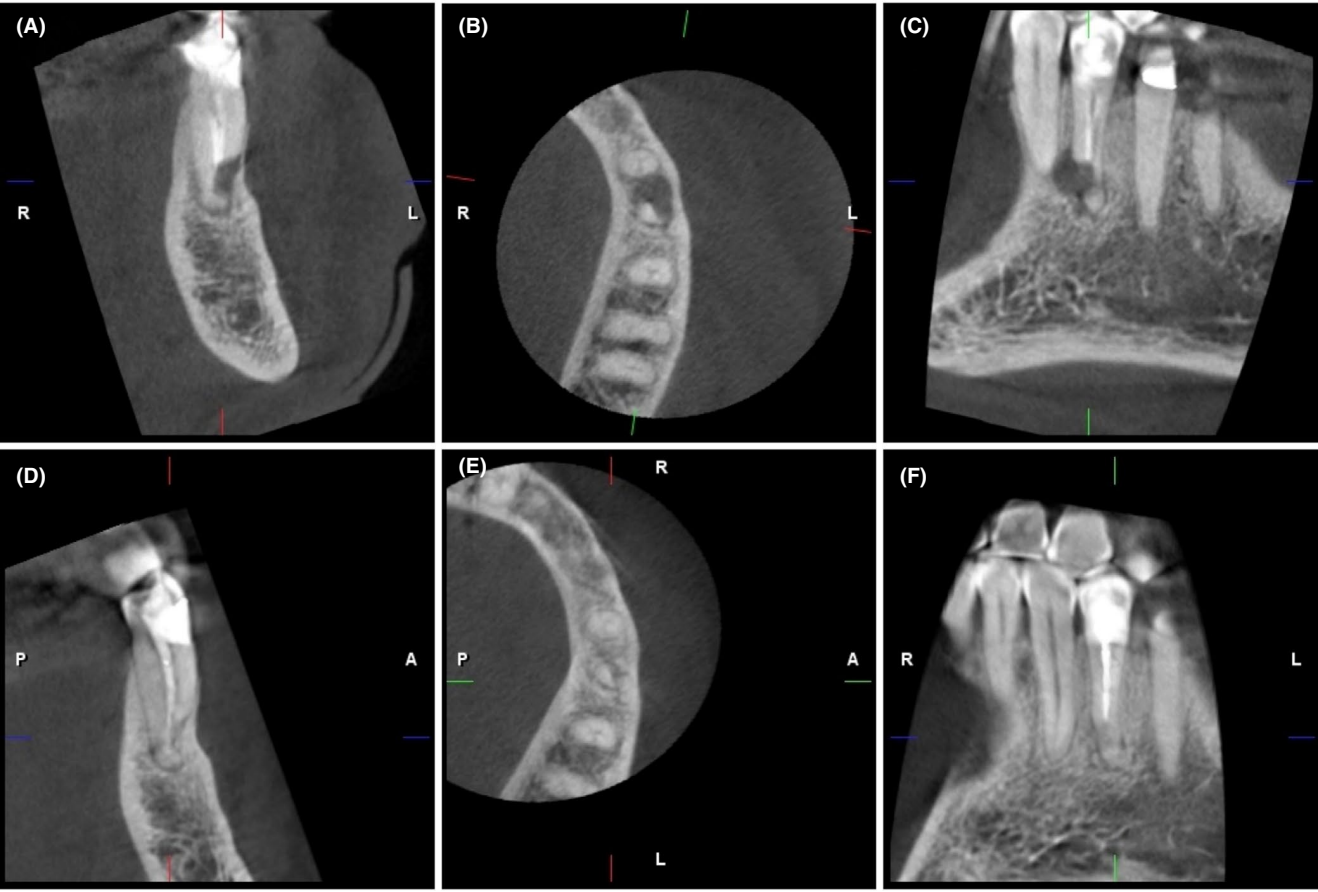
A, B, C, Preoperative coronal, axial, and sagittal CBCT images, and D, E, F, 1‐y follow‐up coronal, axial, and sagittal CBCT images
